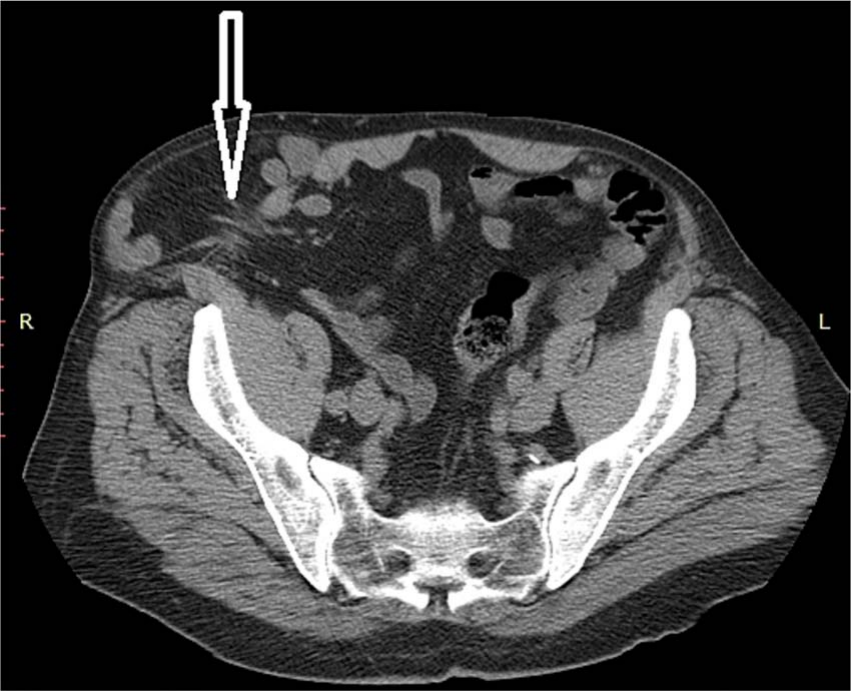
Non-contrast abdominal CT, an arrow pointing to the abdominal wall defect and intestine protrusion
